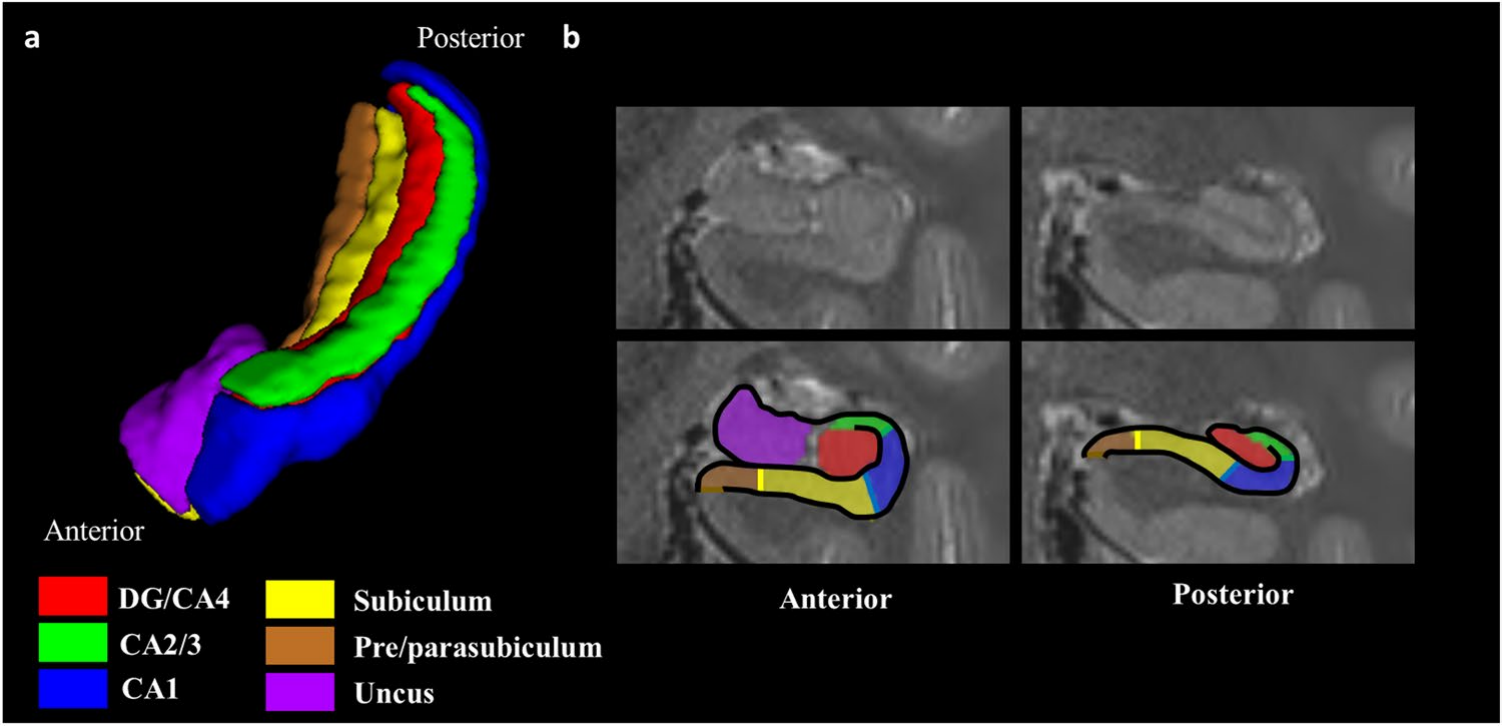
The hippocampus and its subregions. (a) A 3D representation, viewed from an antero-lateral perspective, of the segmented hippocampal subregions. (b) Two sections from T2-weighted structural MRI scans of an example hippocampus (top panel), overlaid with hippocampal subfield masks (lower panel). The left image is from the anterior hippocampus, and the right image from the posterior hippocampus. DG = dentate gyrus, CA = Cornu Ammonis.
